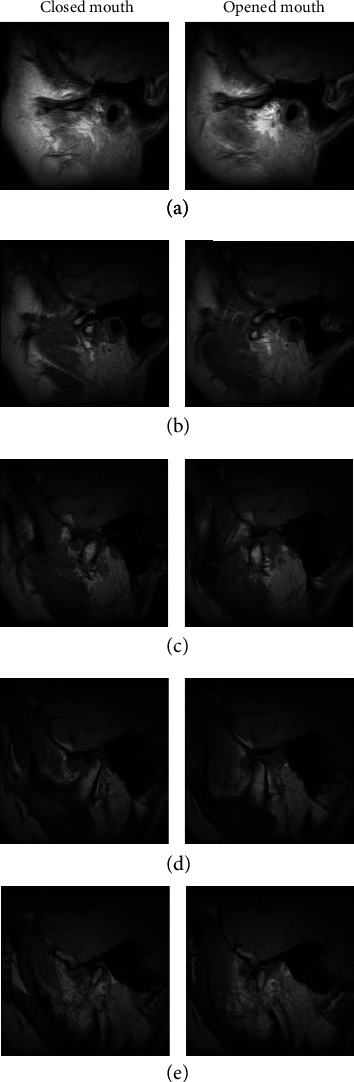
MRI images demonstrating degenerative joint disease in series.
